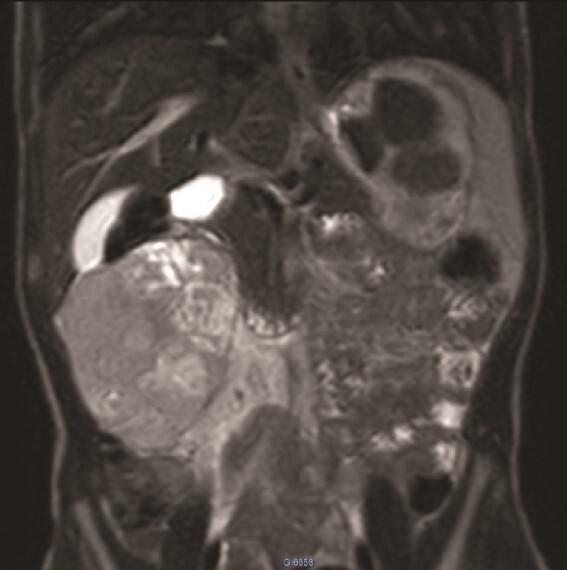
MRI: A solid mass in the upper right of the abdomen without visualization of normal right kidney parenchyma.
